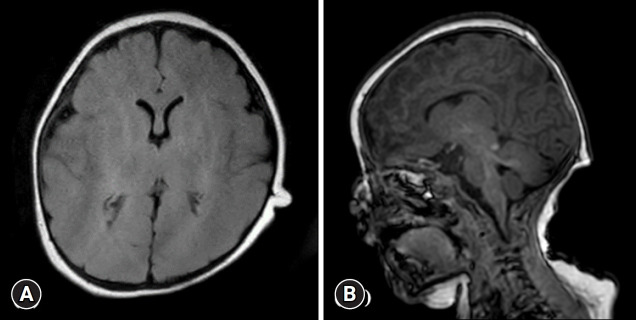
Brain magnetic resonance imaging of the patient. (A) Axial T2-weighted image demonstrates normal cerebral hemispheres and ventricular system. (B) Sagittal T1-weighted image confirms the presence of a normal corpus callosum and other midline structures. No features of holoprosencephaly, ischemic lesion, or hemorrhage are observed.
